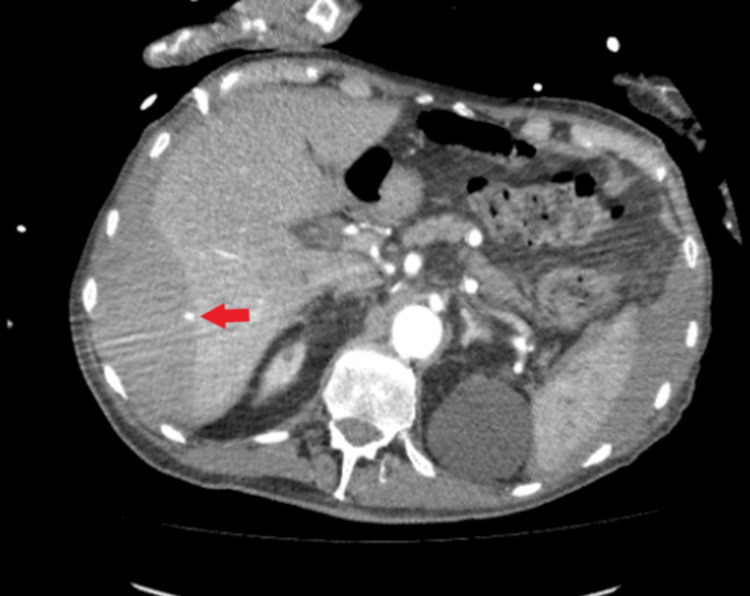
CT angiogram mesenteric artery on Day 50 showing large subcapsular hematoma with a focus of active bleeding There is a focus on arterial extravasation. There is evidence of mild contrast pooling in the venous phase CT: computed tomography
